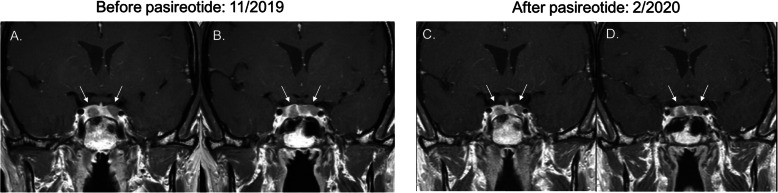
Coronal T1-weighted MRI scans. a and b. November 2019: Bilateral pituitary lesions measuring 14 mm and 7 mm indicative of recurrence of Nelson’s syndrome. Pasireotide was initiated one month after this imaging was completed. c and d. February 2020: Bilateral pituitary lesions measuring 13 mm and 6 mm after 2 months of pasireotide treatment
